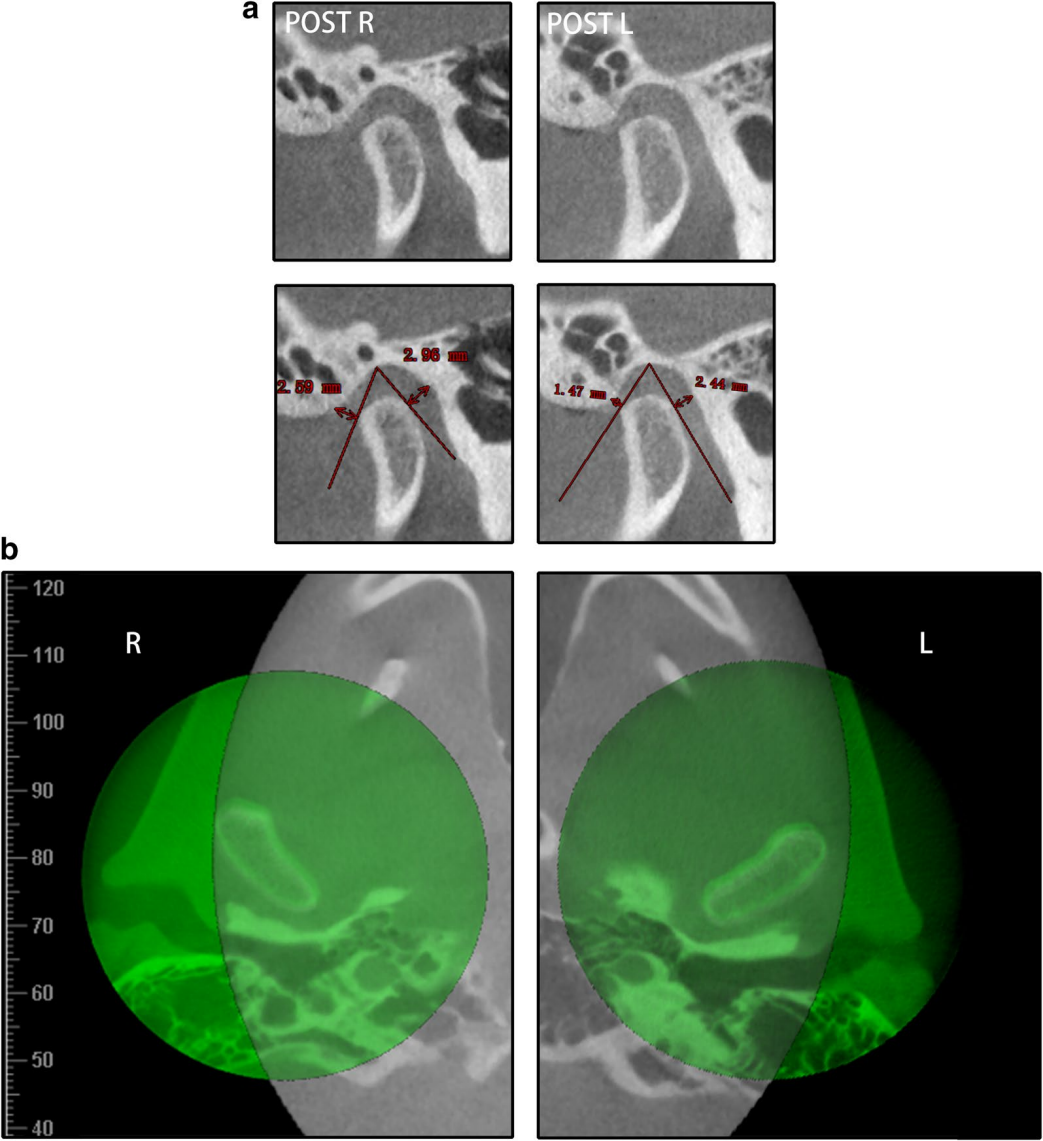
a The post-treatment bilateral TMJ JSIs. b CBCT superimposition of the pretreatment (gray) and post-treatment (green) bilateral TMJs
